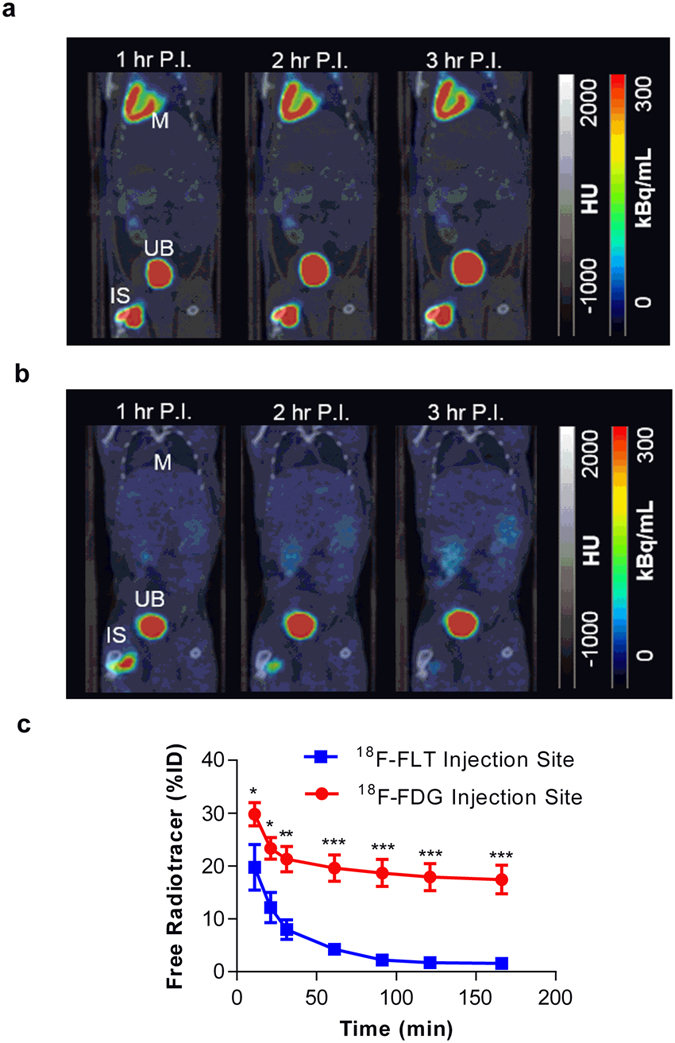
Comparison of free ^18^F-FDG and ^18^F-FLT signal profiles. Representative averaged (1 hr) images from each hour post-injection (P.I.) of (a) free ^18^F-FDG- and (b) free ^18^F-FLT. IS = injection site, M = myocardium and UB = urinary bladder. (c) Free ^18^F-FDG and ^18^F-FLT time activity curves at the injection site, p* < 0.05, *p* ≤ 0.01, *p ≤ 0.001 for ^18^F-FDG- vs. ^18^F-FLT, two-way ANOVA with post-hoc Bonferroni’s multiple comparisons test, n = 3.
